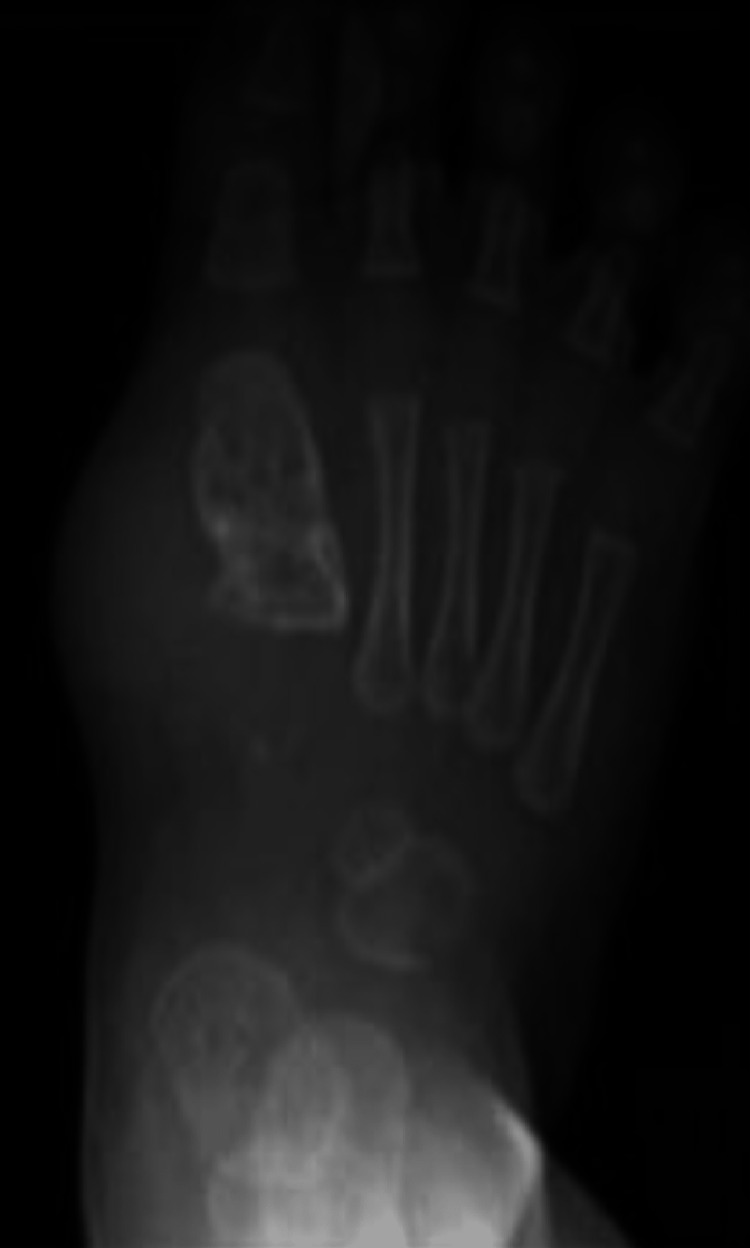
Plain radiograph of the right foot showing soft tissue swelling over the medial aspect of the right foot with multiple lytic areas in the first metatarsal bone with cortical breakthrough
